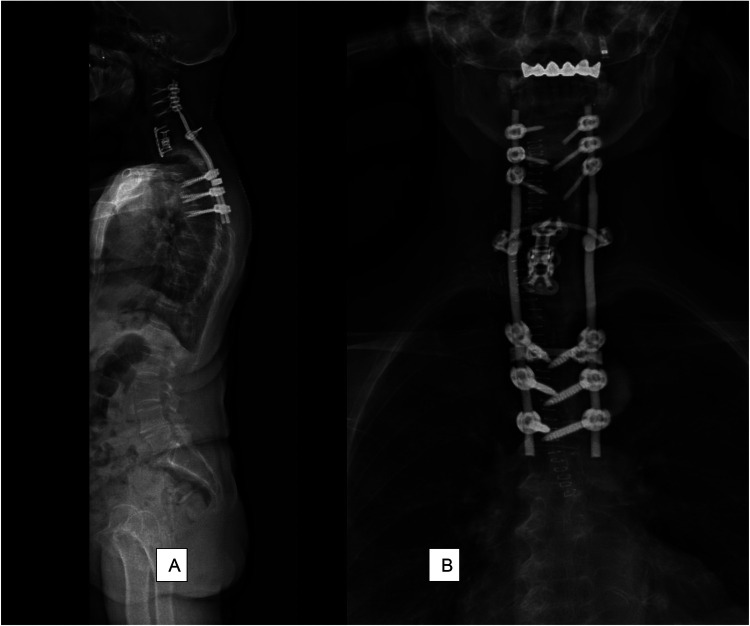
Postoperative standing X-rays. The first surgical stage comprises the extension of the posterior hardware from C4 to T6. Two cervicothoracic rods are used to connect the cervical and thoracic pedicle screws with two cross-links to enhance the strength of the construct. (A) Sagittal plane X-ray; and (B) coronal plane X-ray.
